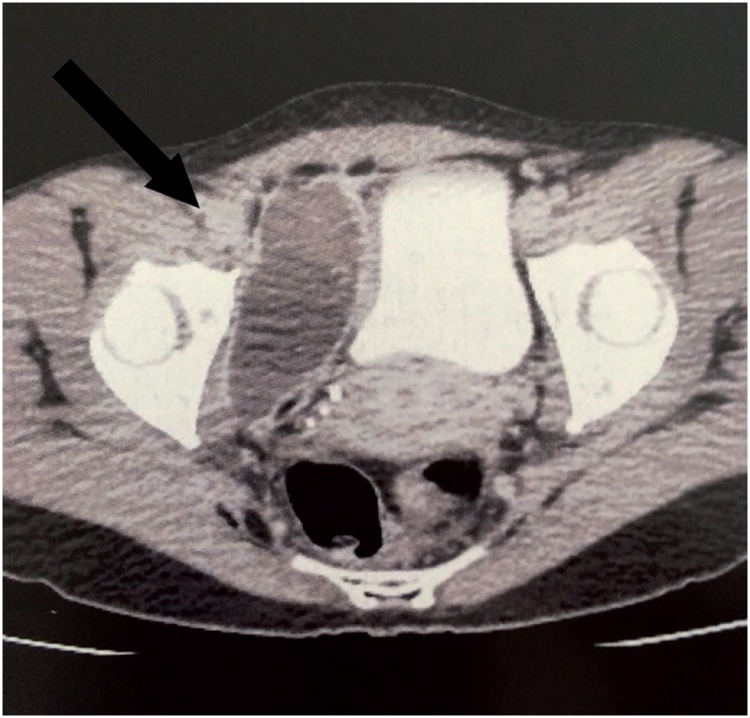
CT scan in the first postoperative day following a retropubic SSS demonstrates large pelvic hematoma (arrow) compressing the bladder laterally.
